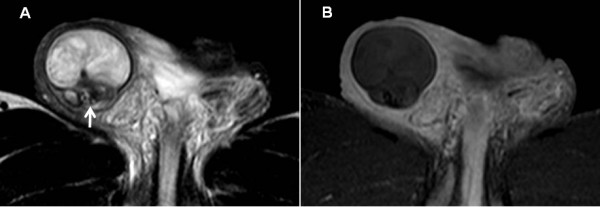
Magnetic resonance imaging of the right testis. (A) T2-weighed image showing that intratesticular intensity was slightly high and a serpiginous vessel (arrow). (B) Dynamic contrast-enhanced subtraction, showing that the intratesticular space had a lack of enhancement.
